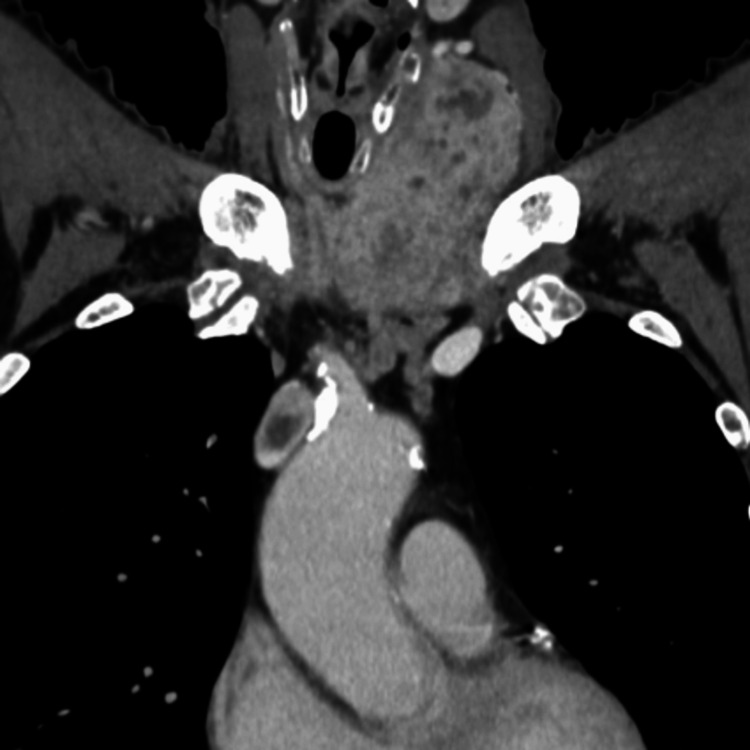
Contrast-enhanced CT showing a tumor measuring 84 × 62 × 54 mm that extends from the left thyroid lobe into the isthmus.
